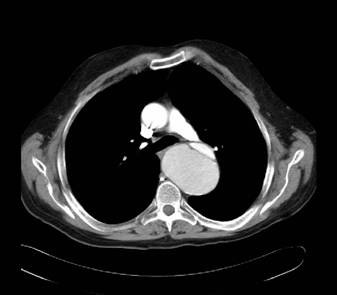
CT scans demonstrating the dissection at the level of the aortic valve.
